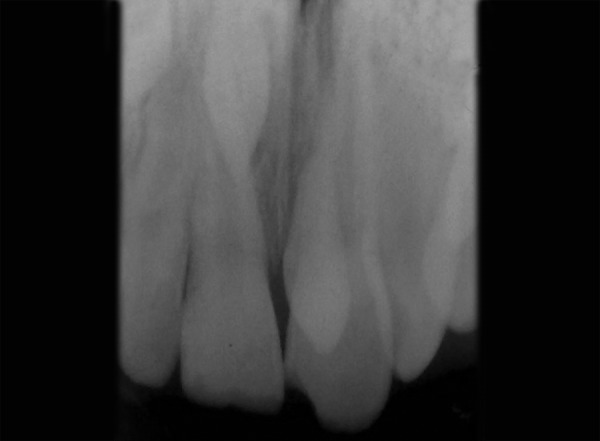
Intraoral periapical radiograph revealing two supernumerary teeth (case II)
